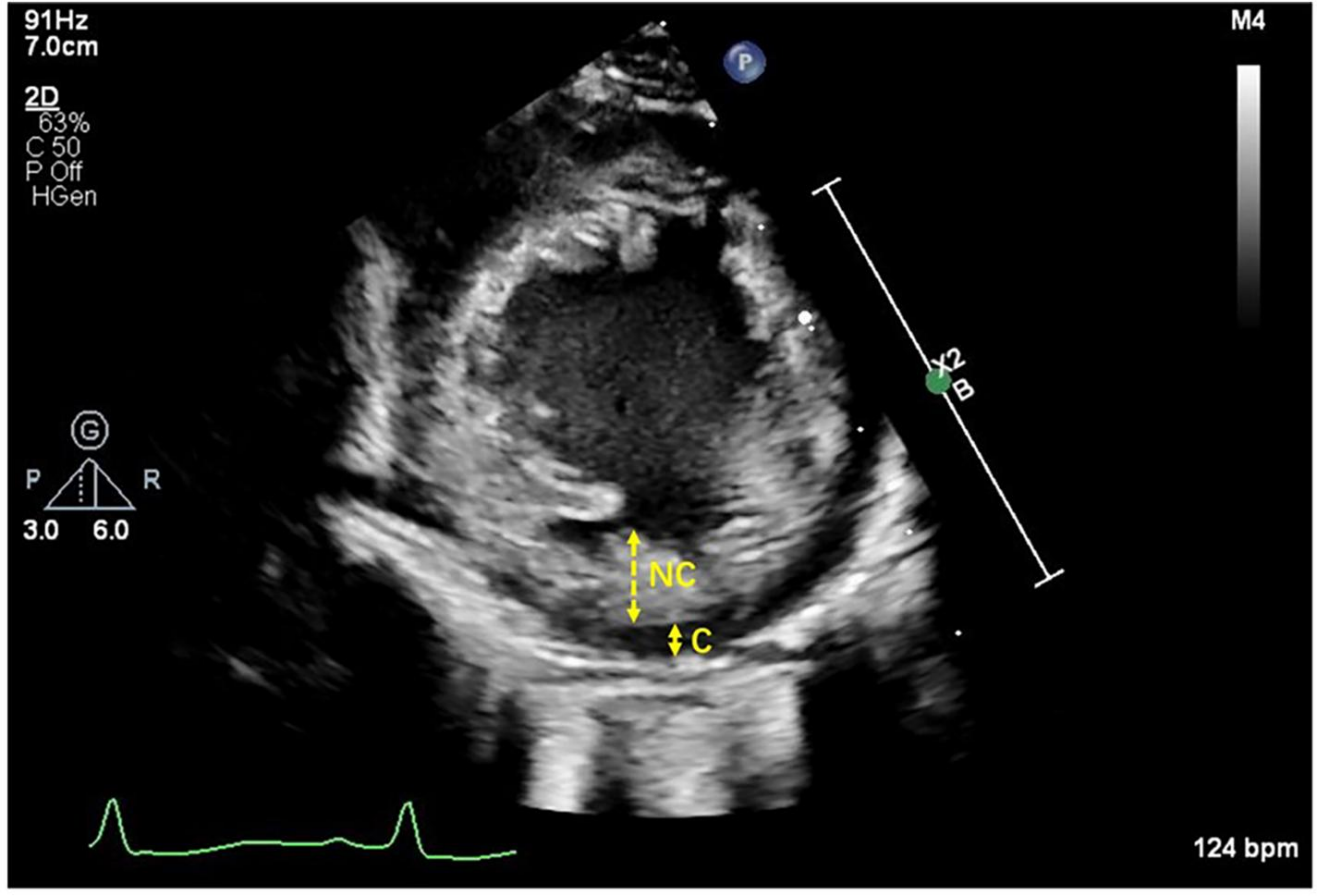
The NC/C ratio was measured in the parasternal short-axis view during end-systole phase. A left ventricular myocardium with two distinct layers, namely, compacted (C) and noncompacted (NC).
